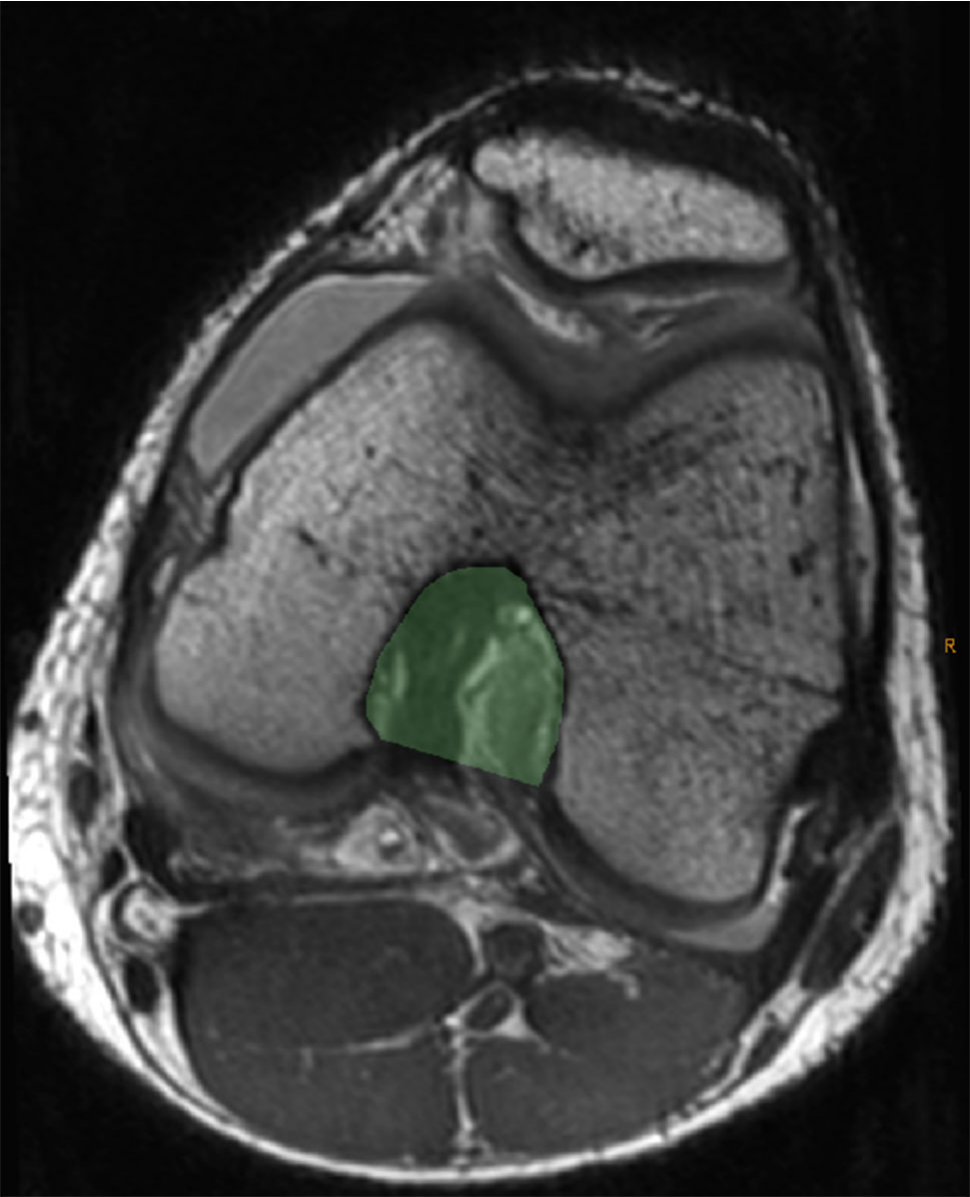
Axial image of a PD MRI sequence demonstrating the measuring technique for the intercondylar notch volume. The measuring technique was applied to all axial images included within the defined borders of the femoral notch and summated to generate the volumetric data
